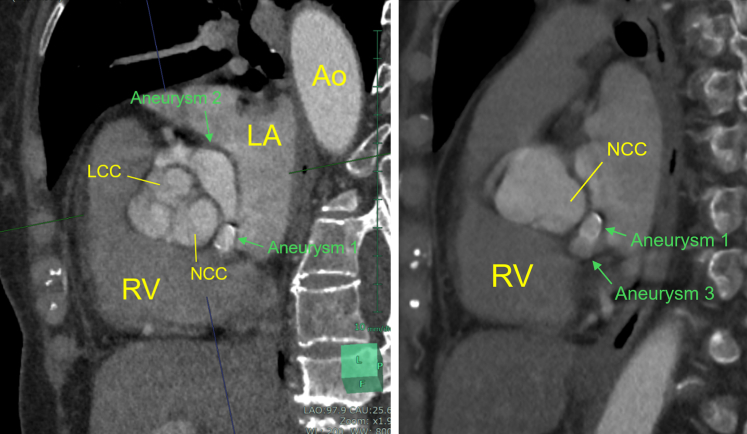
Preoperative Contrast-Enhanced CT The first aneurysm was a 10-mm-diameter aneurysm adjacent to the NCC (Aneurysm 1). The second aneurysm measured 14 mm in diameter and extended toward the LCC (Aneurysm 2). The third aneurysm measured 10 mm in diameter, was continuous with the first aneurysm, and extended toward the left ventricular outflow tract (Aneurysm 3). Ao = aorta; LA = left atria; LCC = left coronary cusp; LV = left ventricle; NCC = noncoronary cusp; RV = right ventricle.
